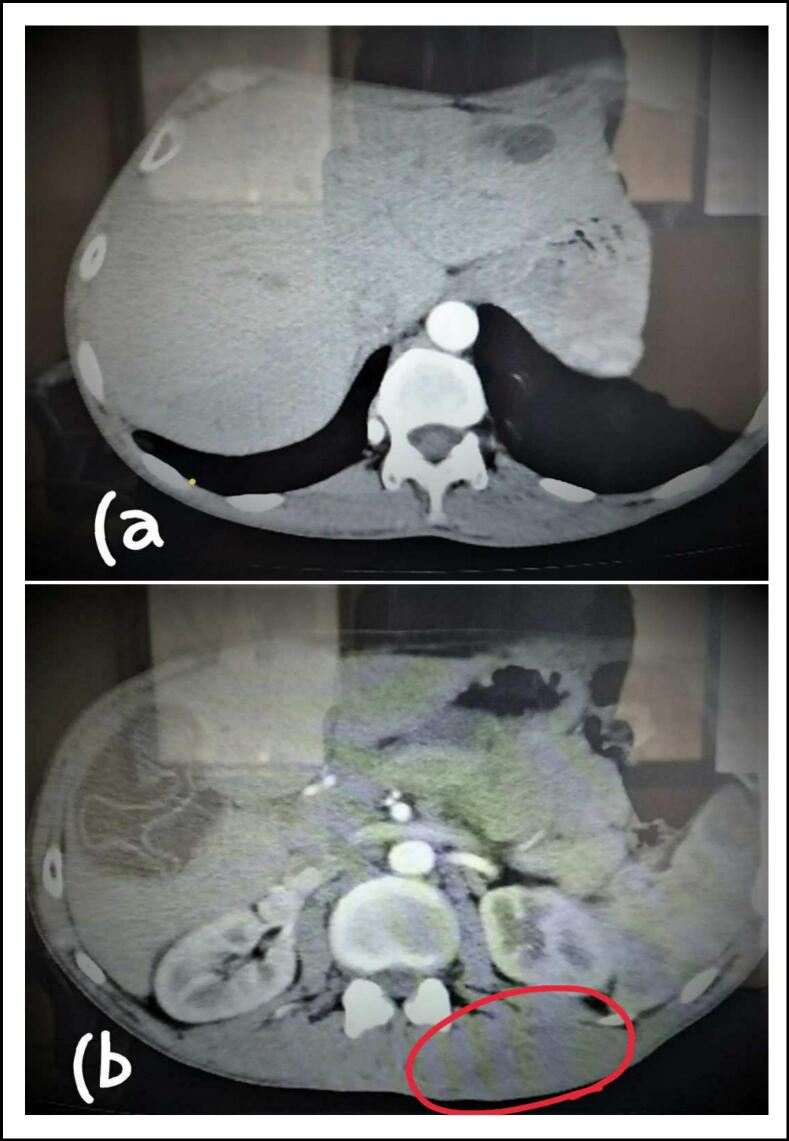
Multislice CT reveals: (A) the cyst in the left lobe that has reduced to 1 cm. (B) The cyst in the right lobe and regression of the cyst in paraspinal muscles on the left side (red circle). (For interpretation of the references to colour in this figure legend, the reader is referred to the web version of this article.)
